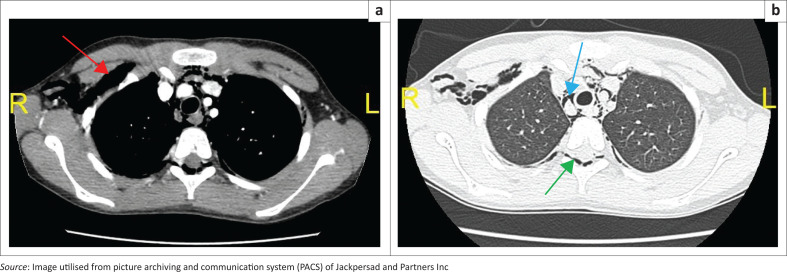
(a and b) Axial post contrast (CT) scan, soft tissue and lung settings, respectively, demonstrating subcutaneous emphysema (red arrow), pneumomediastinum (blue arrow) and epidural emphysema (green arrow).
